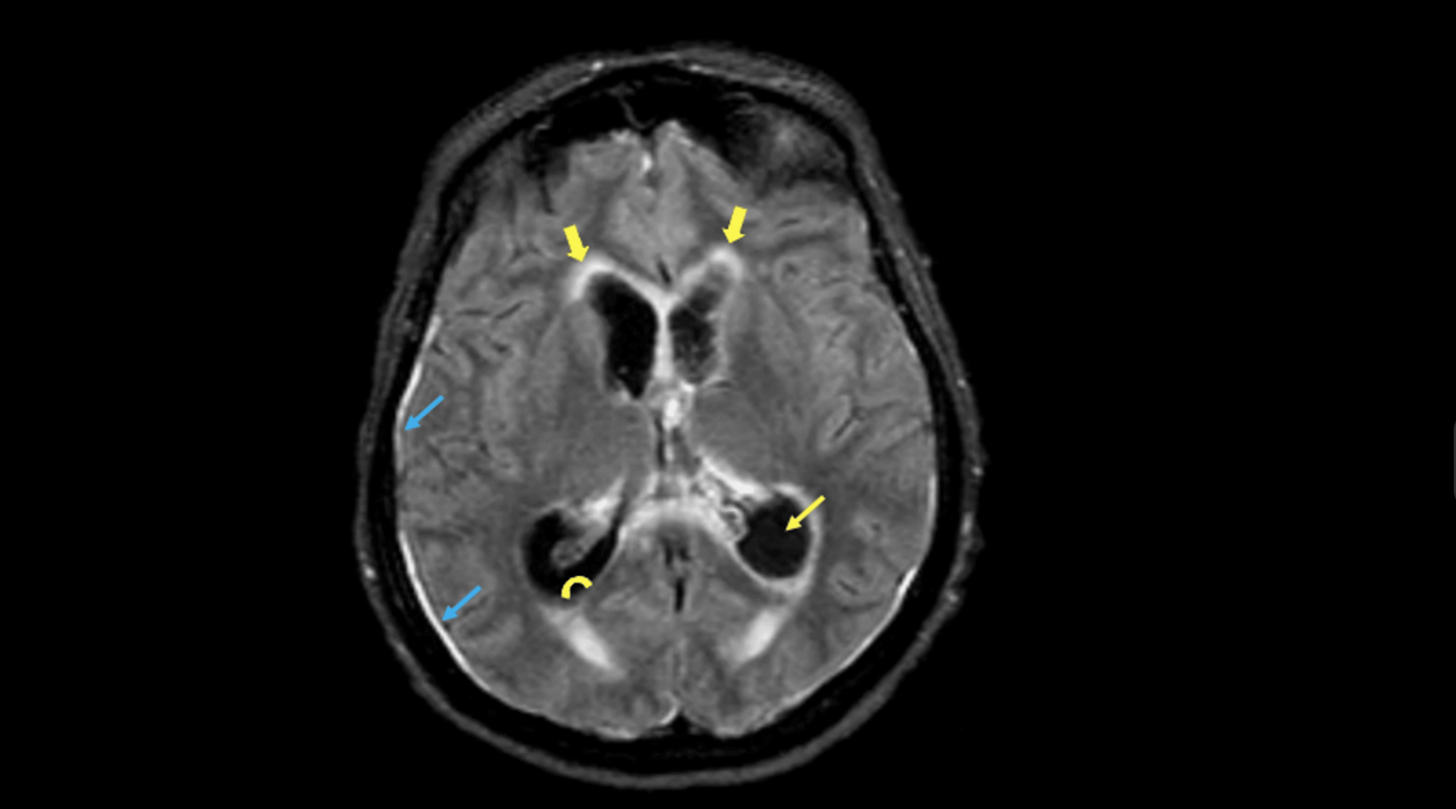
Imaging features of meningitis and ventriculitis on axial FLAIR MRI. Shows diffuse periventricular FLAIR signal (thick yellow arrows), hydrocephalus (thin yellow arrow), layering debris (curved yellow arrow), and meningeal hyperintense signal (blue arrows). FLAIR: fluid-attenuated inversion recovery.
